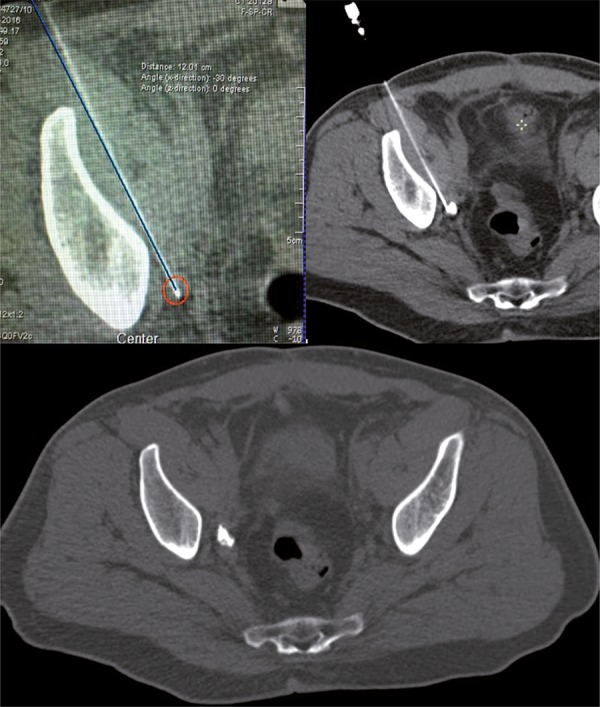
CT-GUIDED PERCUTANEOUS PUNCTURE OF THE LYMPH NODE USING A EXTRAPERITONEAL LATERAL APPROACH 20G NEEDLE AND 3ML OF SOLUTION OF 4% COLLOIDAL CHARCOAL AND LIPIODOL WAS INJECTED INTO THE LN.
